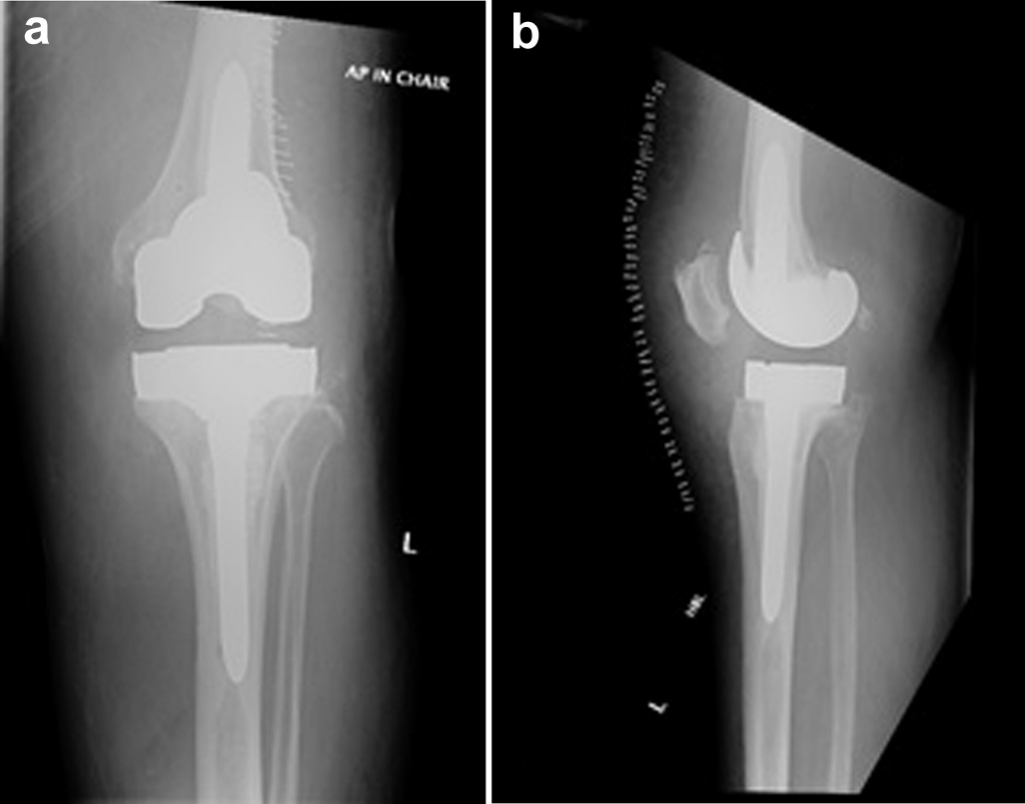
(a) Anteroposterior and (b) lateral radiographs immediately after revision TKA.
